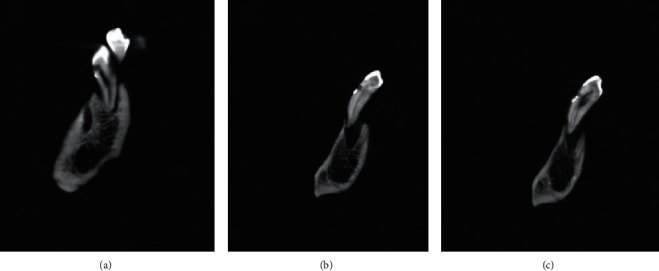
Marginal leakage on the gingival wall detected by the Planmeca CBCT unit (Planmeca ProMax 3D Mid machine, Helsinki, Finland). (a) Score 0. (b) Score 1. (c) Score 2.
